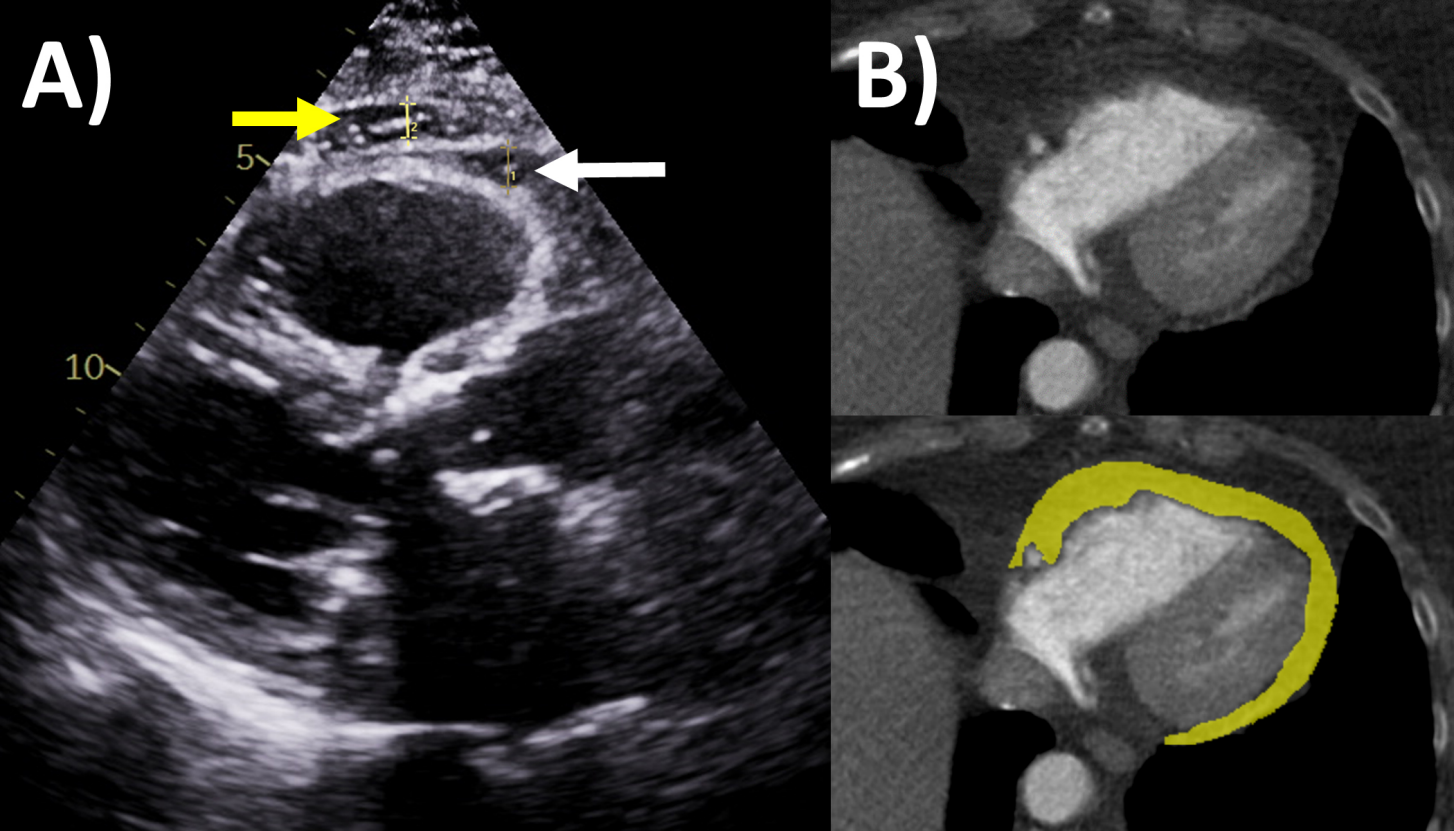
Epicardial fat evaluation by echocardiography and computed
